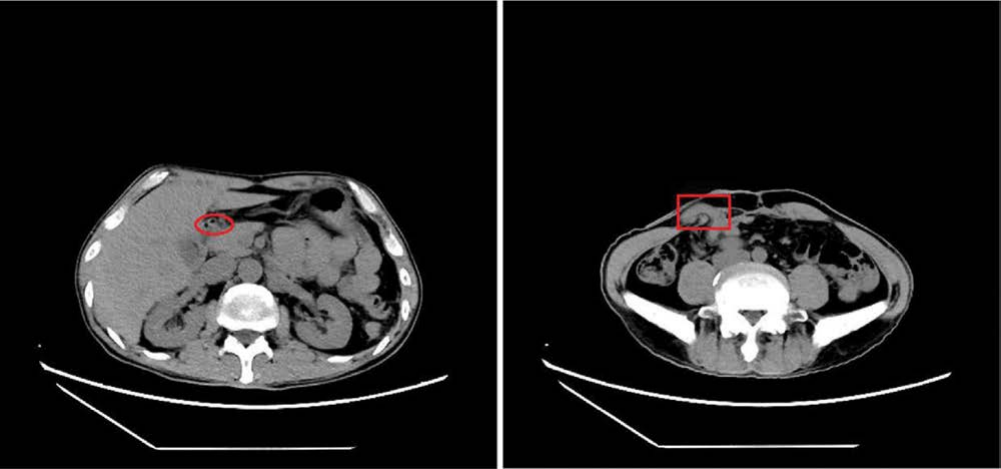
Abdominal CT findings. Red circle: intra-abdominal free gas. Red rectangle: small intestine overflowing the abdominal wall.
